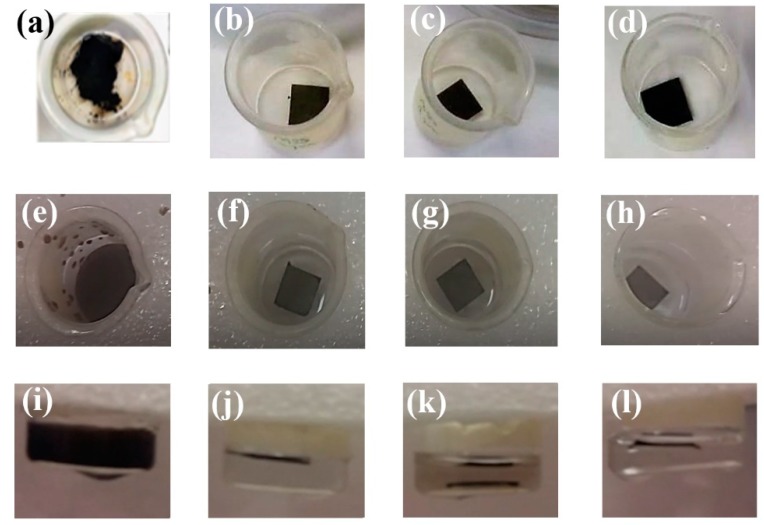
(a,e,i) GO, (b,f,j) 25GO/N, (c,g,k) 50GO/N, and (d,h,l) 75GO/N membranes in distilled water: (a–d) static mode, (e–h) top view, and (i–l) cross view after ultrasonication.
